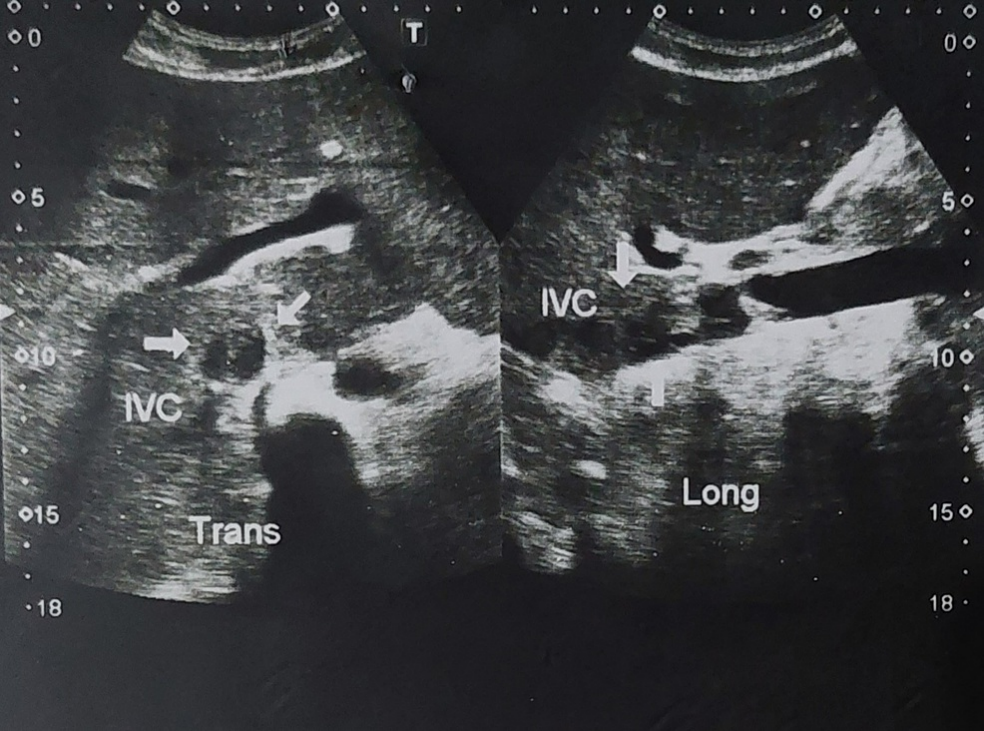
Ultrasound abdomen and pelvis showing inferior vena cava thrombus
